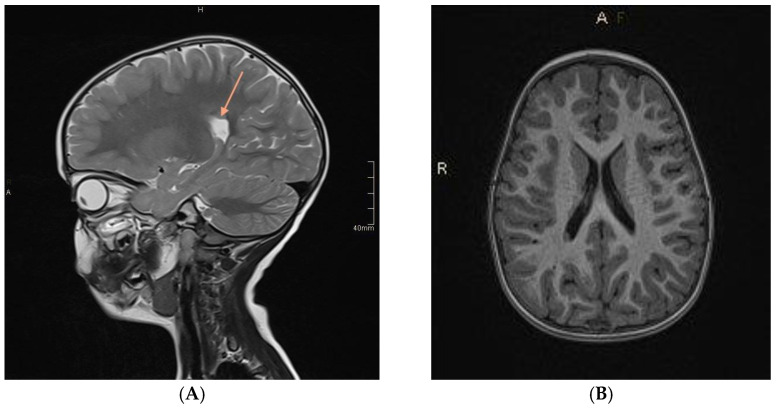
Head MR imaging (T2−weighted (A) and T1−weighted (B) images) of a 3−year−old boy after the third episode of seizures. MR examination showed a single focus of heterotopia. Source: own study.
